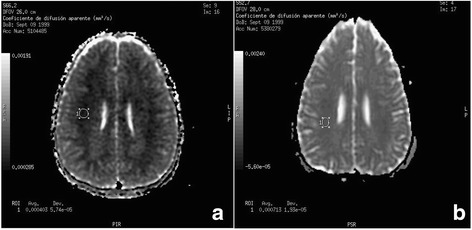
Diffusion-Weighted Imaging in MRI, comparing the ADC map, at 72 h (Image a) and after 8 months (Image b). It may be observed that the circular region of interest to determine the ADC value in both images: with values of: 0,4 × 10^−3^ mm^2^/s at 72 h (restricted diffusion), whilst after eight months the value was 0.7 × 10^−3^ mm^2^/s (normal ADC map value)
